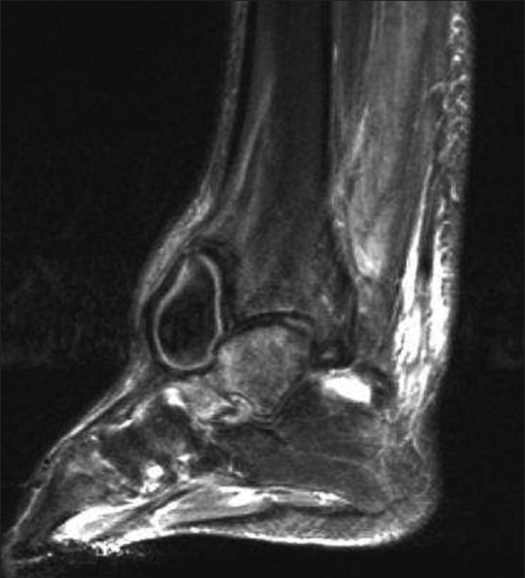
Sagittal MRI of the right ankle confirming the diagnosis of a pseudoaneurysm arising from the anterior tibial artery as it crossed the ankle joint
